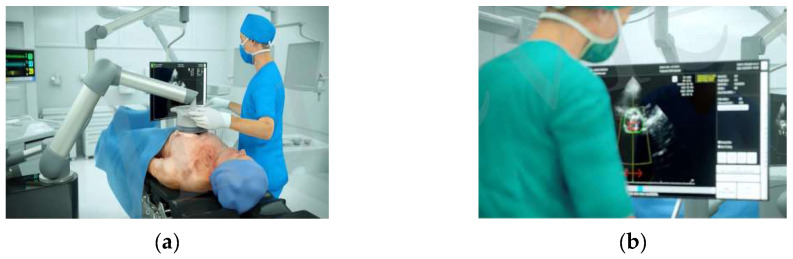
Valvosoft device using non-invasive ultrasound therapy. During treatment, the applicator, which contains an imaging ultrasound and an independent therapeutic transducer, is positioned against the patient’s chest (panel (a)). The aortic valve is targeted and imaged using 2-D echo live monitoring (panel (b)). The image was reproduced with the permission of Cardiawave, Levallois-Perret, France.
